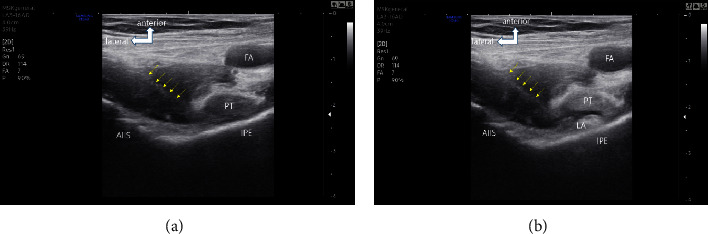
Ultrasound images of pericapsular nerve group block performed via a lateromedial approach. (a) Shows the needle position. The needle is outlined by yellow arrows. (b) Shows the local anesthetic spread following injection. FA, femoral artery; LA, local anesthetic; IPE, iliopubic eminence; AIIS, anterior inferior iliac spine; PT, psoas tendon.
